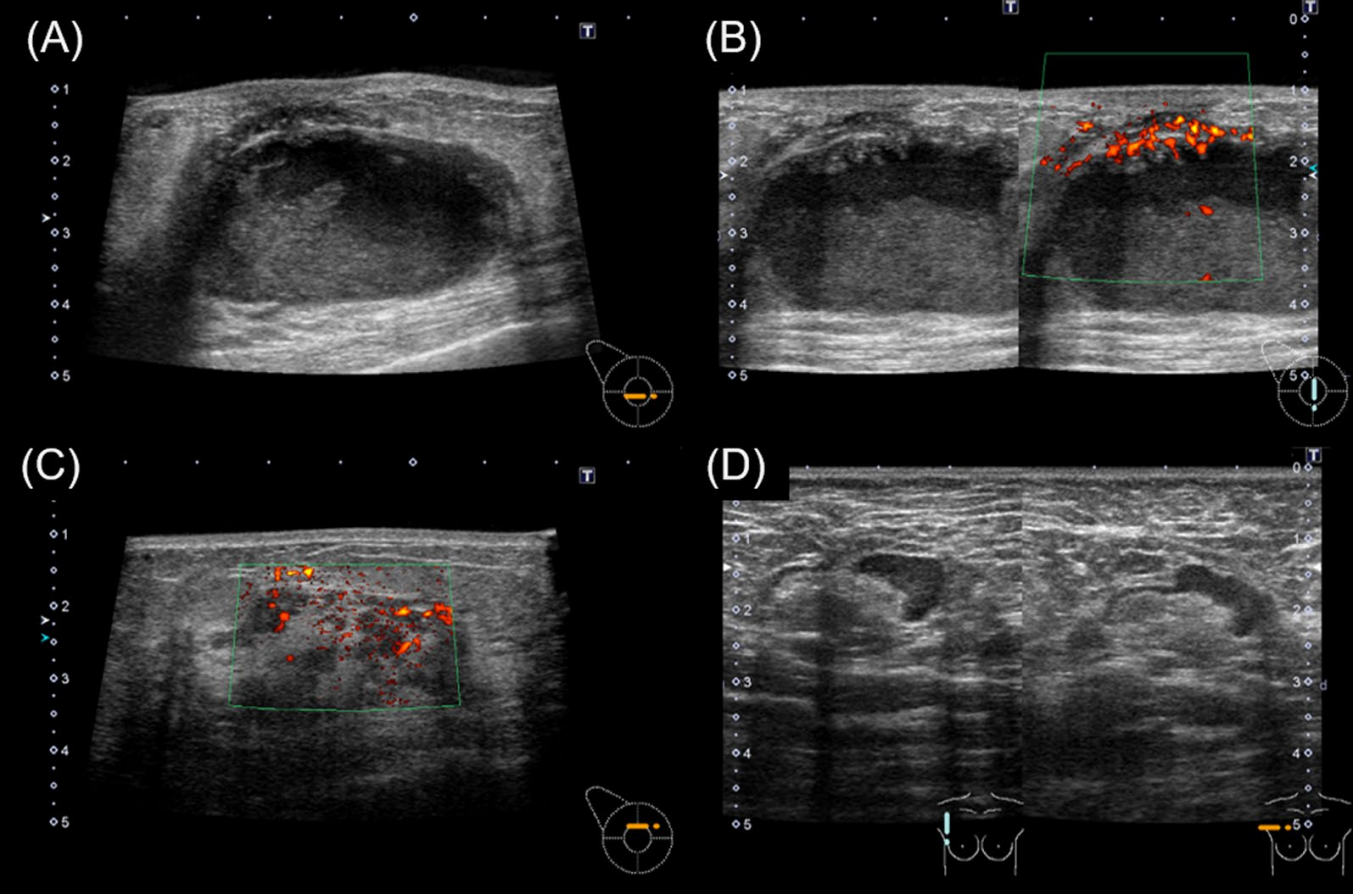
Findings on ultrasound.
